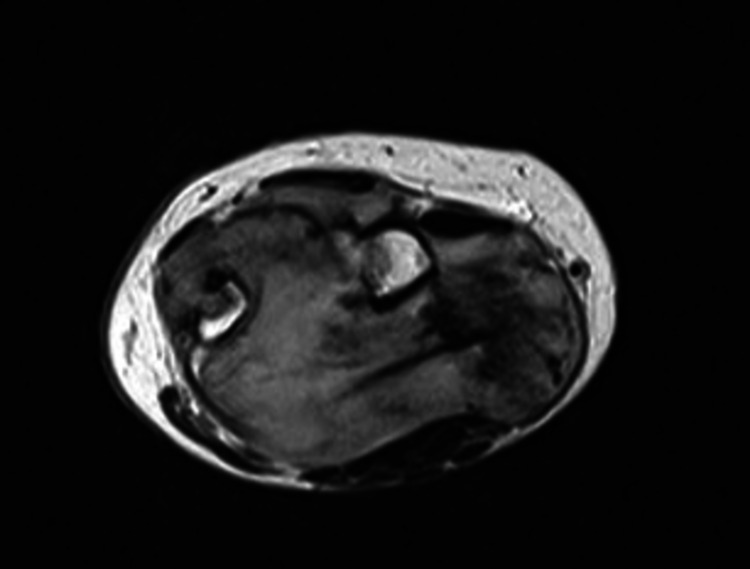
Axial view of the left wrist (MRI; T1W image). The mass shows an increased and heterogenous signal density. MRI: magnetic resonance imaging; T1W: T1-weighted
